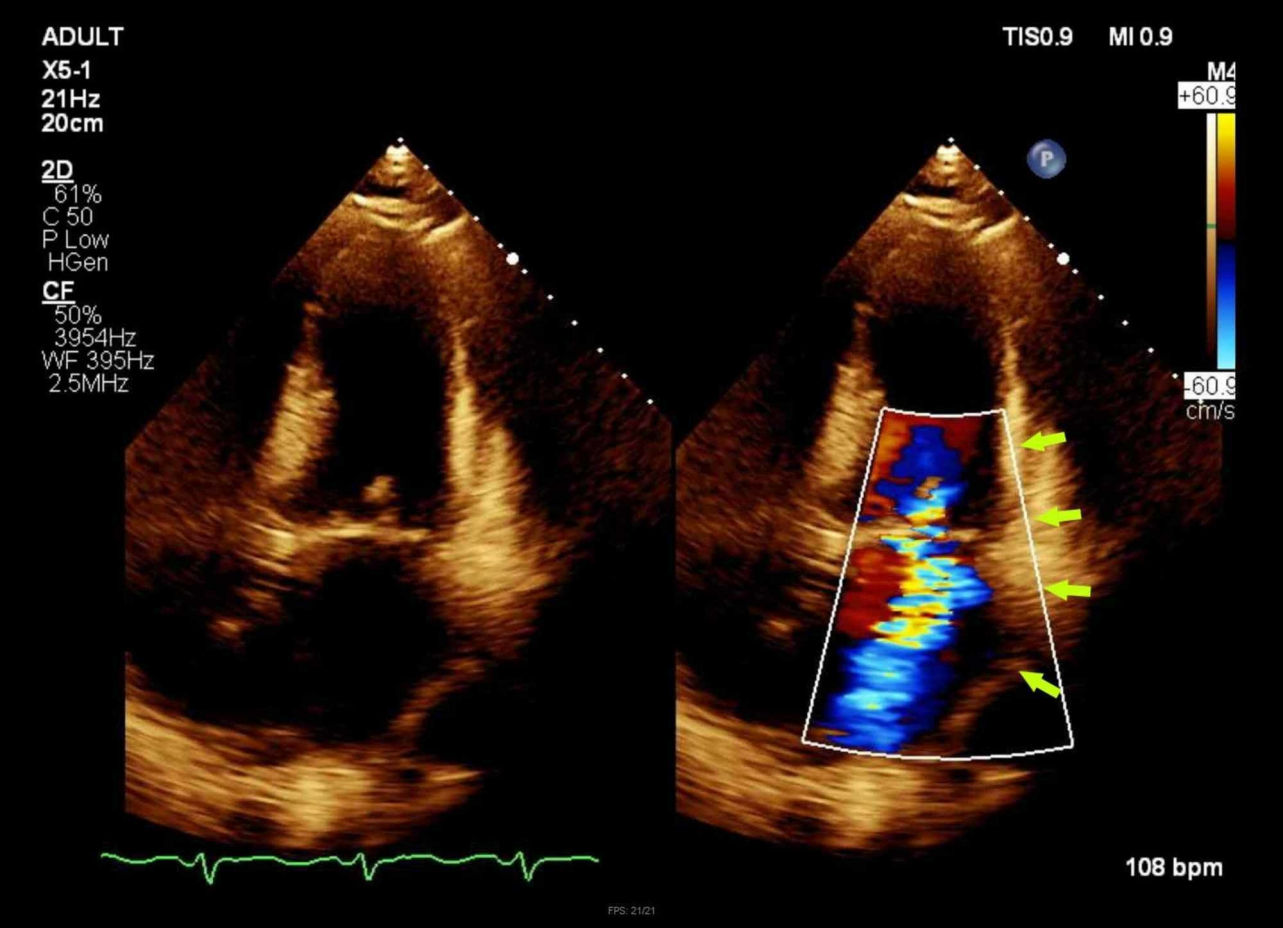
Transthoracic echocardiogram (TTE) revealing severe mitral regurgitation on Doppler flow (arrows)
